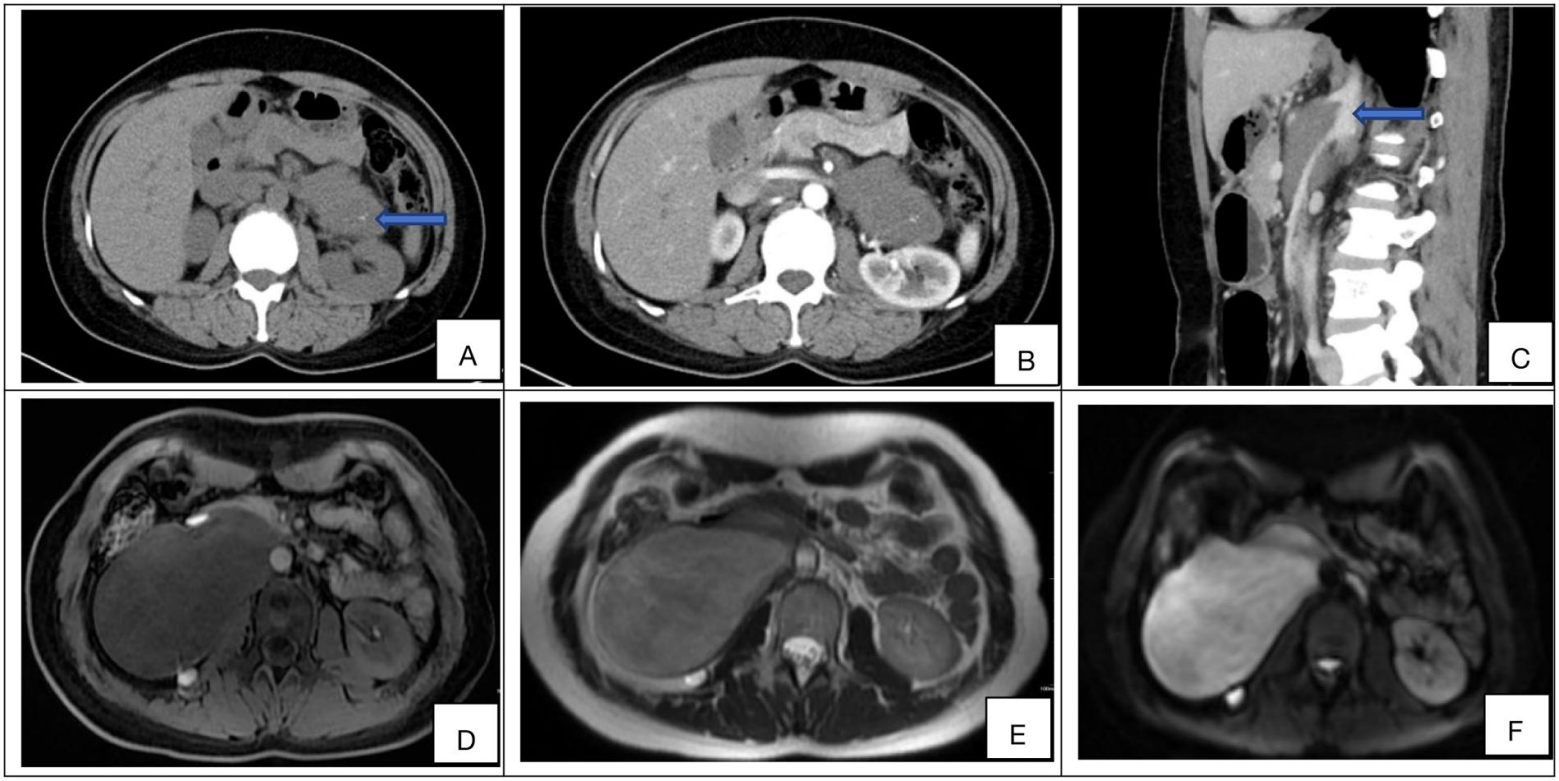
(A–F) Representative preoperative imaging findings of retroperitoneal ganglioneuroma. (A) Axial non-contrast CT demonstrates a hypoattenuating mass (mean density: 32.63 HU) with internal curvilinear calcifications (blue arrow). (B) Axial contrast-enhanced CT (portal venous phase) shows minimal intralesional enhancement (ΔHU = +6.85) relative to muscle. (C) Sagittal non-contrast CT reveals circumferential encasement of the celiac trunk and superior mesenteric artery without luminal stenosis (blue arrow). (D) Axial T1-weighted MRI (non-contrast) displays low signal. (E) Axial T2-weighted MRI demonstrates heterogeneous hyperintensity with internal septations. (F) Diffusion-weighted imaging (DWI) shows high intensity, and the compared sequences show varying degrees of enhancement (from none to strong).
